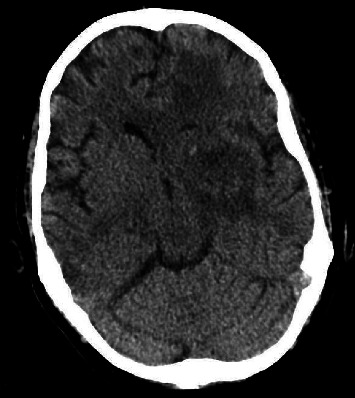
Final CT axial head without contrast showed increased mass effect with decreased attenuation within the left frontal lobe as compared to prior imaging results.
